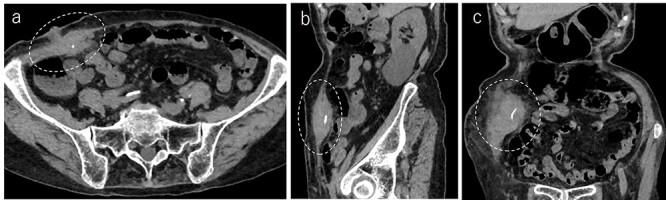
Abdominal CT demonstrated a tumor in the abdominal wall, which extended to the surface of the body (a, encircled); the tumor was 60 mm in width and contained a linear shaped agent; sagittal (b, encircled) and coronal (c, encircled) views.
